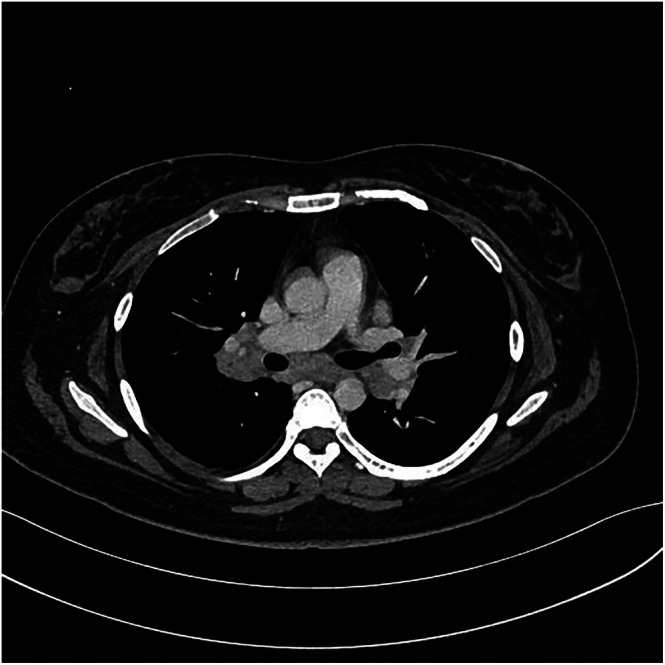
Contrast‐enhanced axial chest computed tomography image on admission. Soft tissue shadows are observed from the mediastinal to the bilateral hilar regions, indicating multiple lymphadenopathy. Findings are predominant on the right side, with some areas of low attenuation suggesting internal degeneration.
